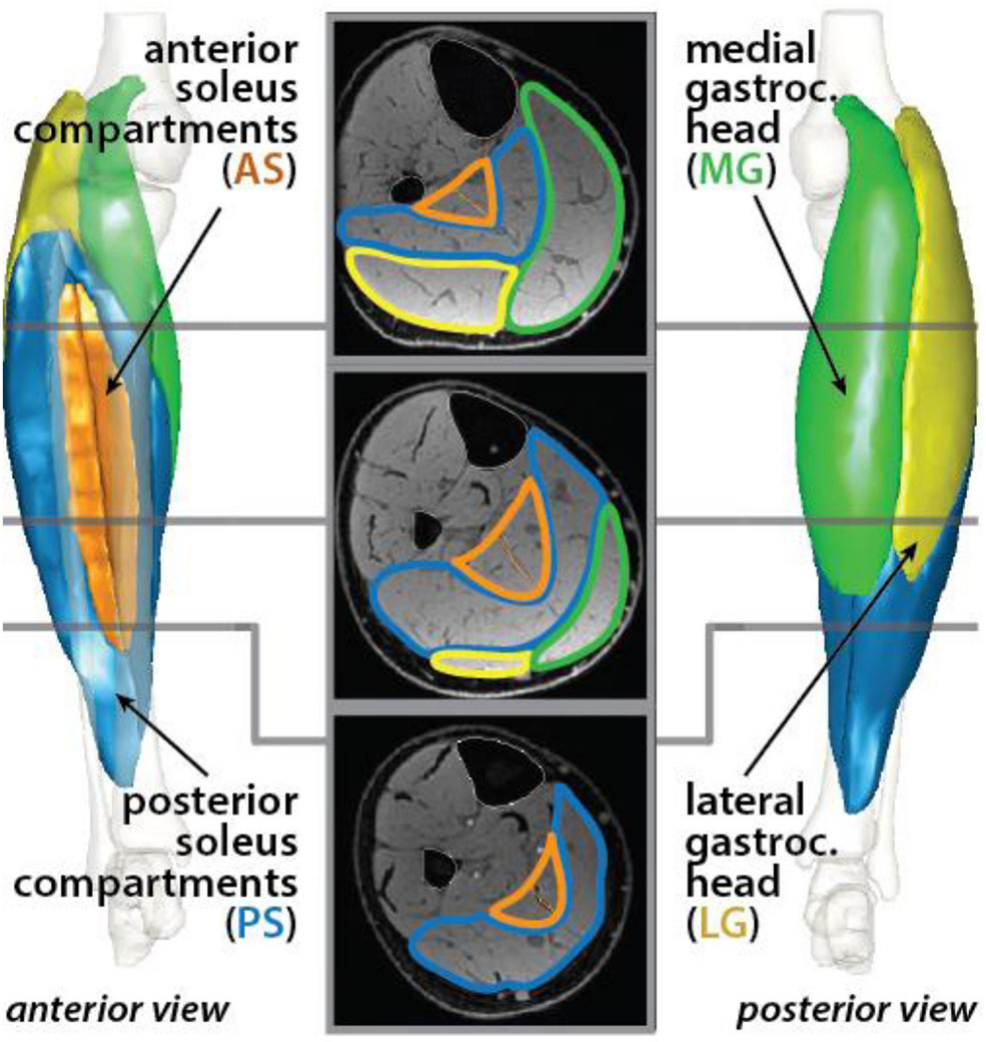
Triceps surae muscles were segmented in axial MR images. The muscle cross sections were defined for the medial (green) and lateral (yellow) heads of the gastrocnemius and the posterior (blue) and anterior (orange) compartments of the soleus. Each muscle was reconstructed in 3-D.
